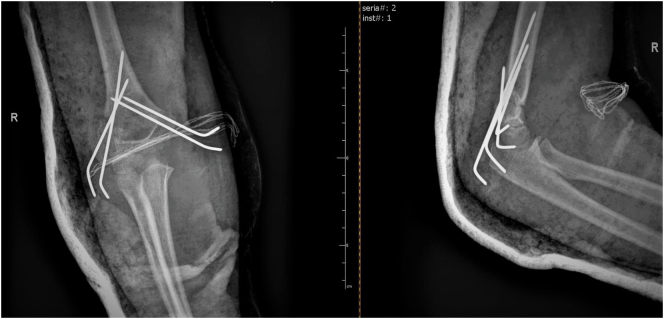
Postoperative radiograph. Open reduction with lateral and medial column pinning. AP and lateral view.
